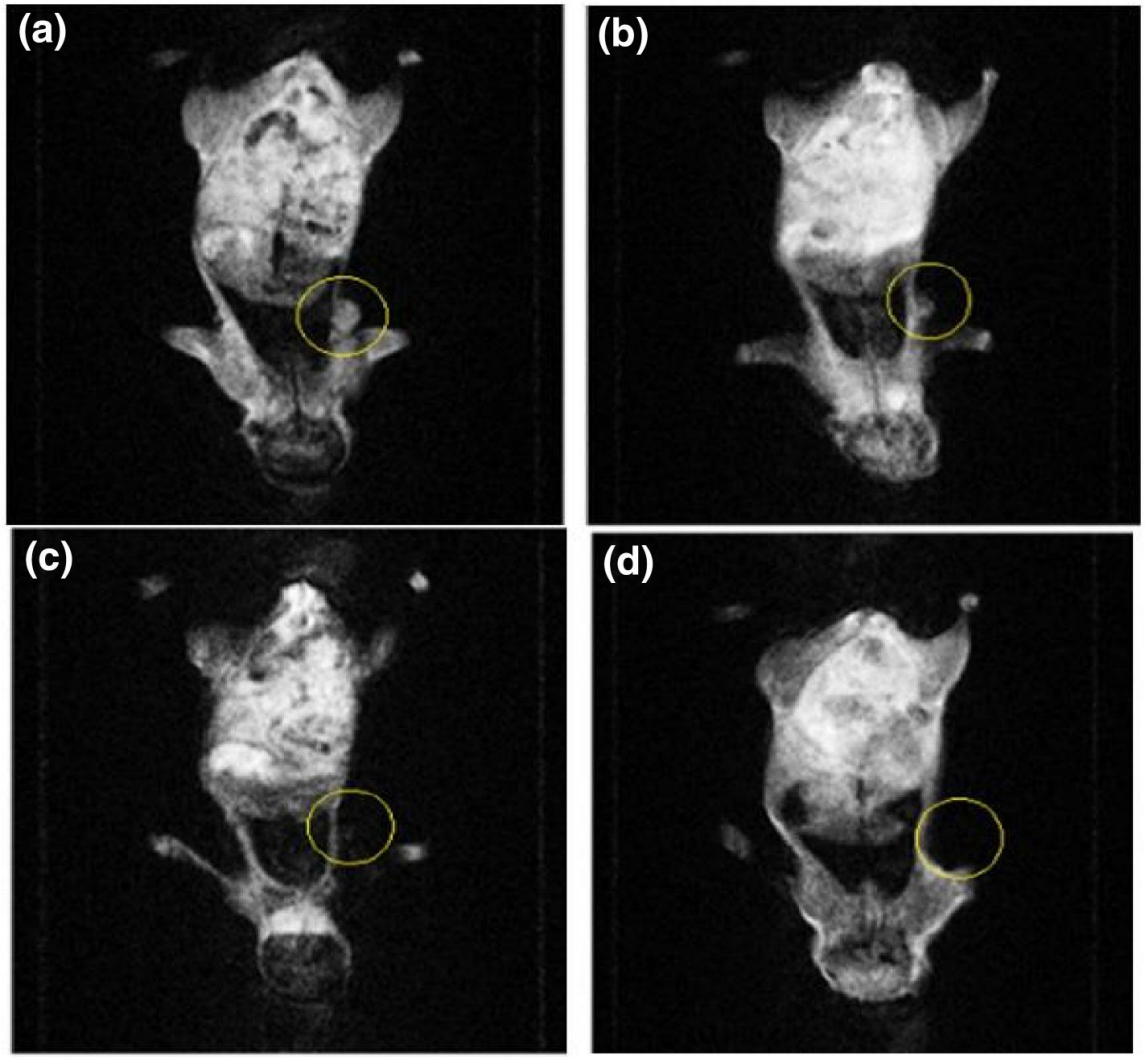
Magnetic resonance imaging of intravenous injection after (a) 0 h, (b) 2 h, (c) 4 h, and (d) 24 h
